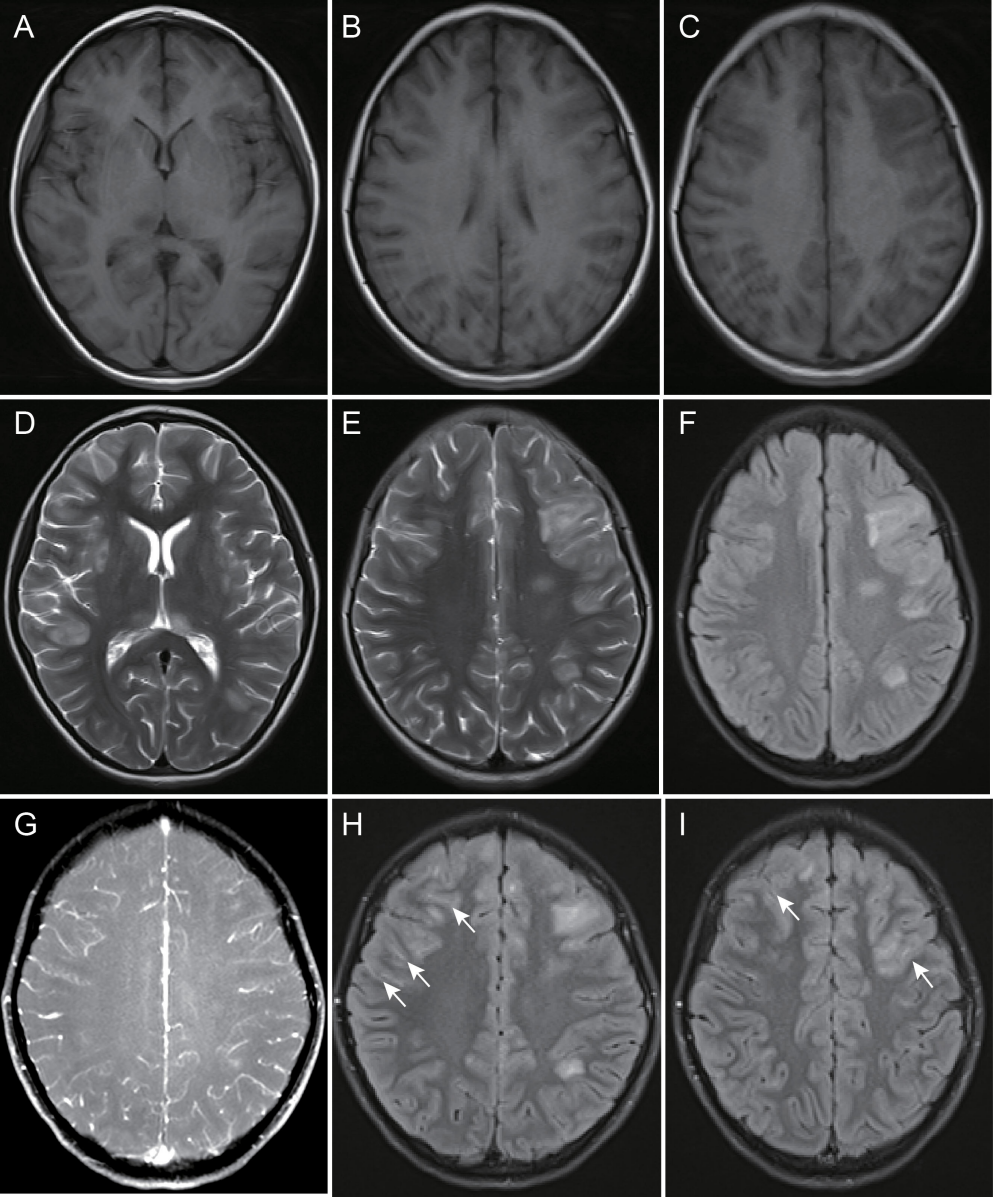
A 142-month-old female child with myelin oligodendrocyte glycoprotein antibody-associated encephalitis had a 10-day history of intermittent fever, which was accompanied by a decreased level of consciousness for 2 days. Magnetic resonance imaging showed multiple abnormal signals and blurred edges in the right basal ganglia, bilateral thalamus, left deep white matter, right temporal lobe, bilateral frontal parietal lobe cortex gray matter, and juxtacortical white matter, hypointensity on T1-weighted images (A-C), and hyperintensity on T2-weighted and fluid-attenuated inversion recovery images (D-F). There was no leptomeningeal enhancement on contrast-enhanced fat-suppressed T1-weighted images (G) and partial leptomeningeal enhancement in bilateral frontal lobe surface on contrast-enhanced fluid-attenuated inversion recovery images (H, I), arrow).
